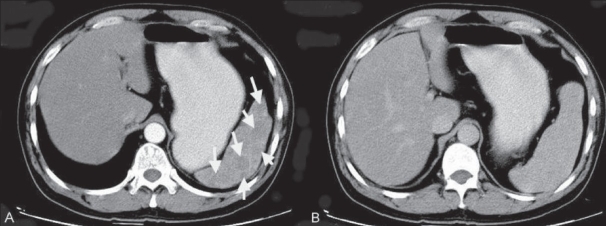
LCA of spleen. Case 2. A 47-year-old Chinese man with multiple splenic lesions. The arterial phase contrast-enhanced CT scan image shows multiple low-attenuation lesions (arrows). The delayed CT scan image (B) shows that the lesions have now become isodense to the adjacent splenic tissue
